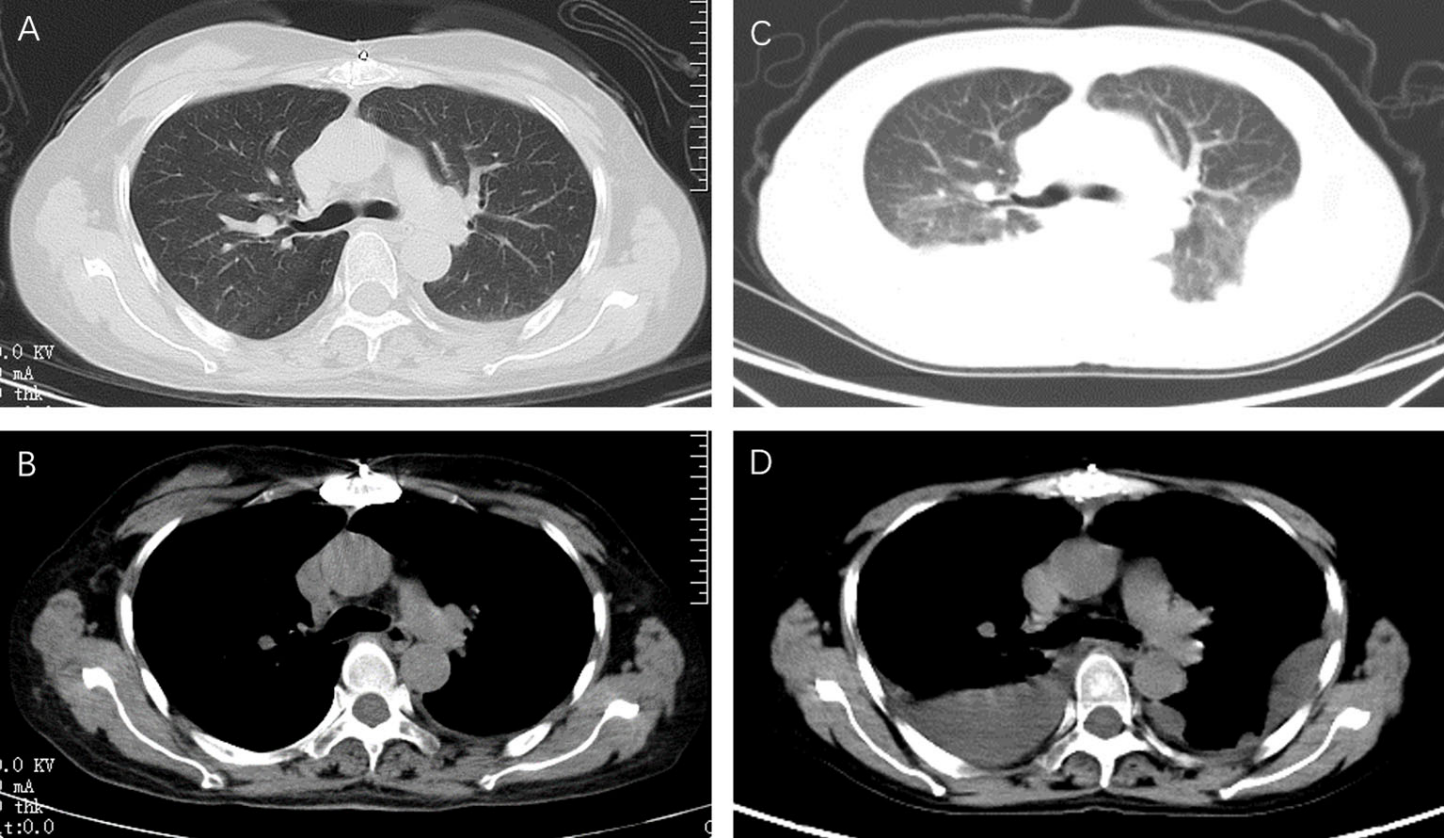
Chest computed tomography at different time points. In the patient with myasthenia gravis after thymoma surgery, a small left pleural effusion was noted on a transverse axial chest CT during regular follow-up (A, B). According to all examinations, the neurologist thought that the chest tightness was due to the recurrence of myasthenia gravis. Methylprednisolone (40mg per day) combined with gamma globulin (400mg per kg) was given. After three days of treatment, the patient's dyspnea worsened. A chest CT was taken again, showed a new bilateral pleural effusion (C, D).
